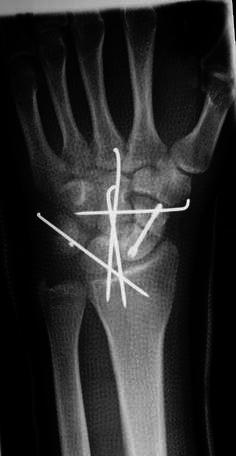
Wrist PA X-ray taken post-operatively
